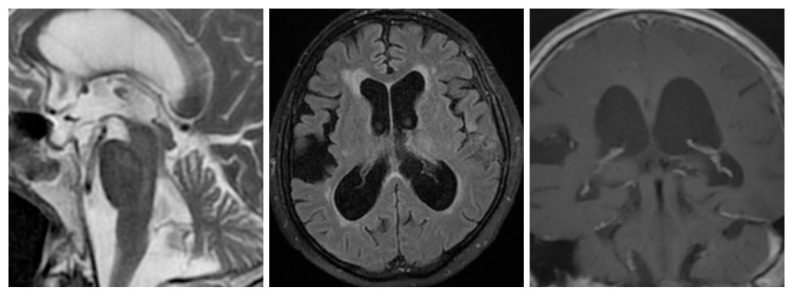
Sagittal T2W image; an iNPH patient the concave appearance of the mesencephalon and vascular compression is shown. 2b. Axial FLAIR image; lateral ventricular dilatation and right-sided transport sulci and periventricular hyperintensities are present. 2c. The coronal image shows the narrow callosal angle and dilated lateral ventricles.
